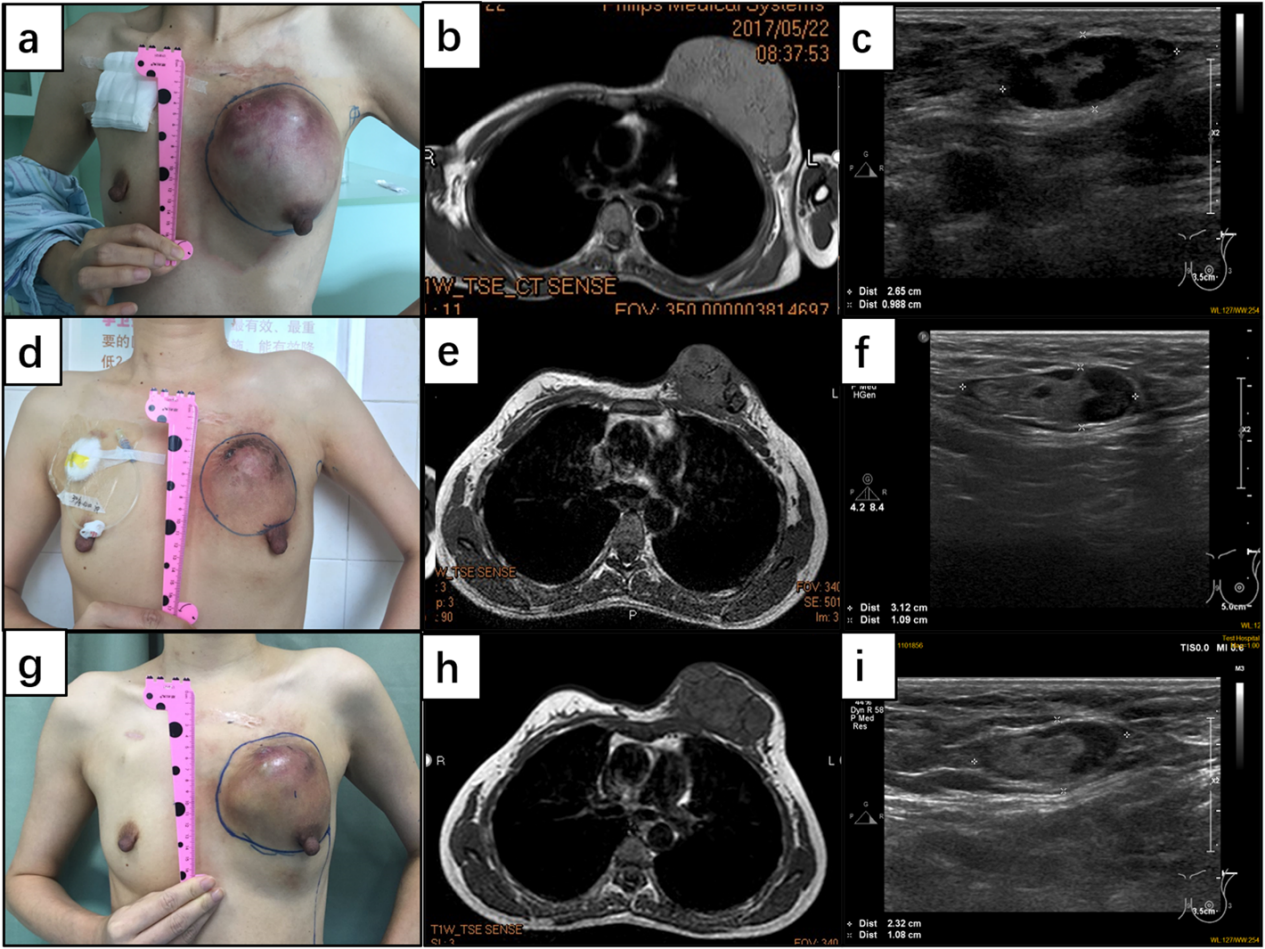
Physical, imaging and ultrasound examination of tumor during NAC. a, b, c: pre-chemotherapy; d, e, f: after 4th cycle of EC; g, h, i: 25 days after 2nd cycle of DC
